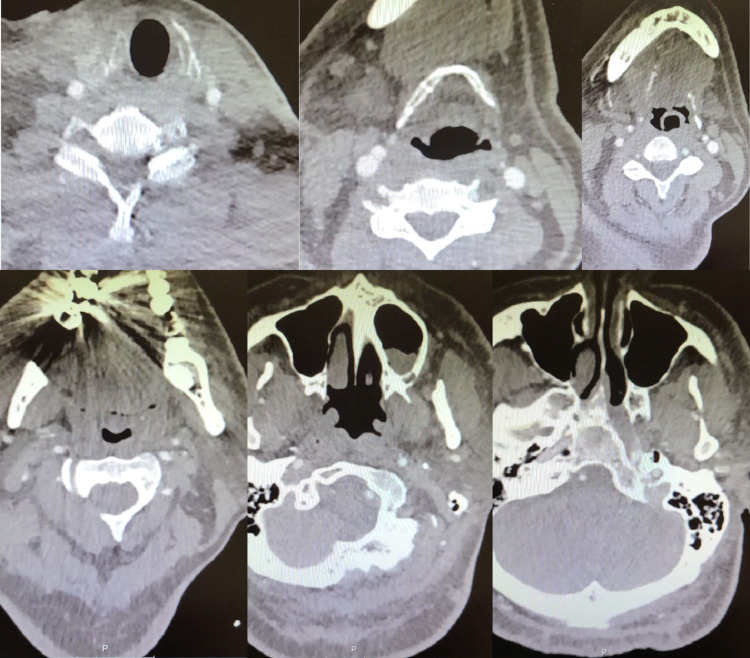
CT angiography of neck and head showing no evidence of hemodynamically significant vertebral arteries stenosis.
